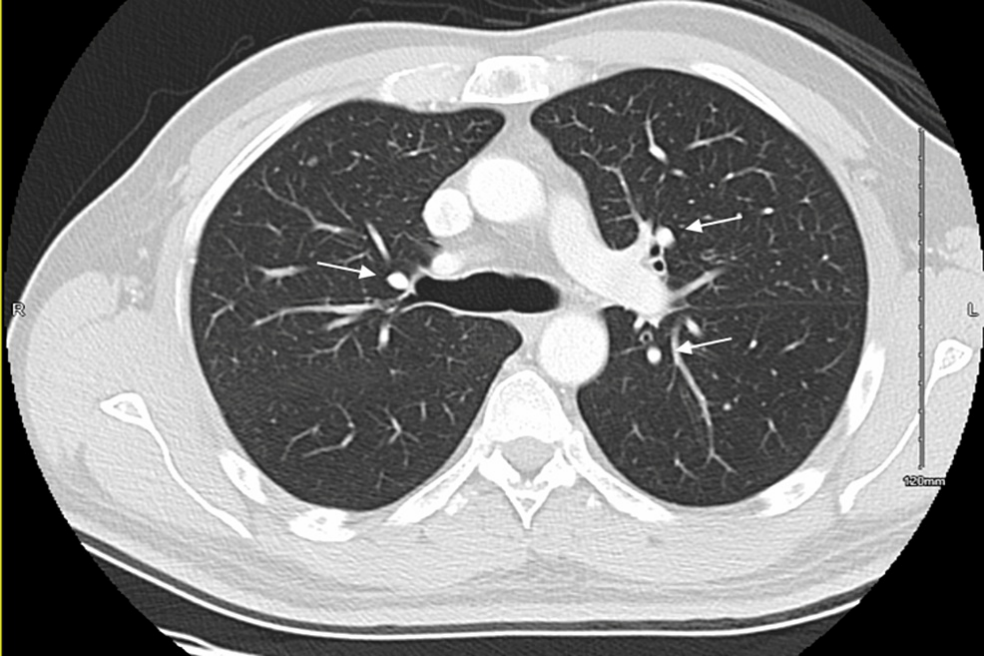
Contrast-enhanced axial CT of the chest. Contrast-enhanced axial image of the chest demonstrates a dominant nodule in the right upper lobe, mostly metastasis. Other nodule characteristics are not suspicious, and they could be inflammatory nodules.
